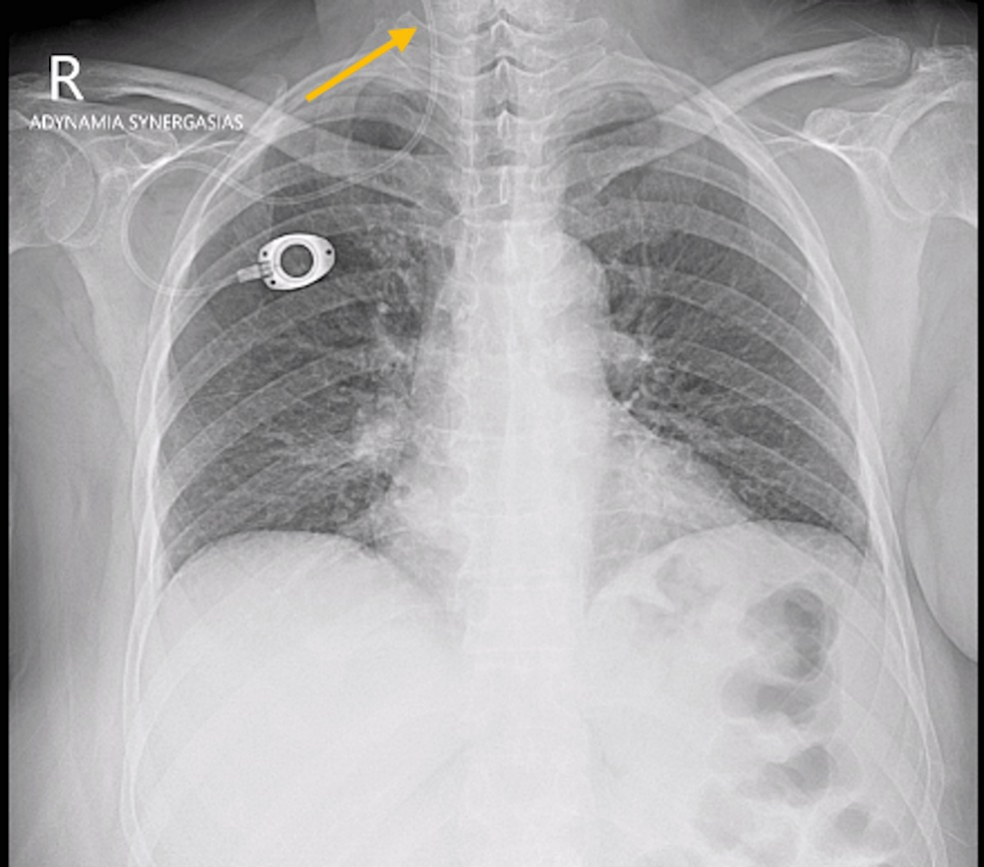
Chest X-ray showing the distal tip of the catheter into the right internal jugular vein
